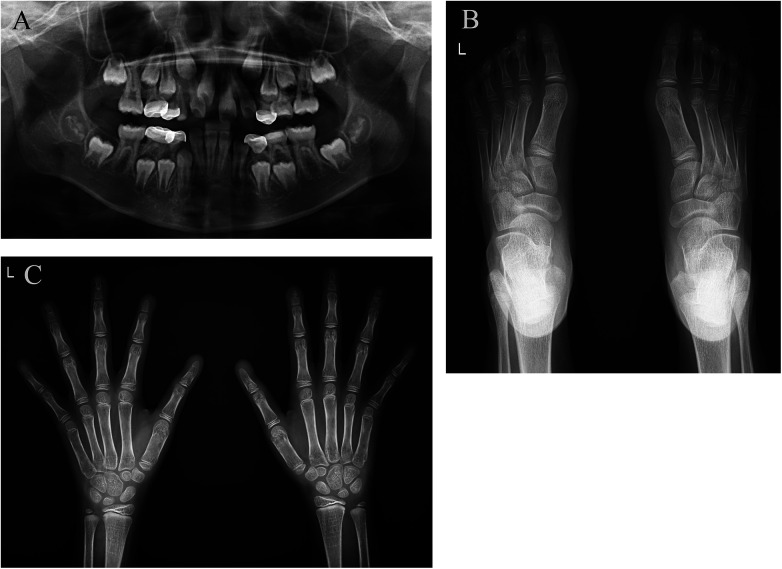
x-ray examination of the patient's teeth, hands, and feet. (A) Shows dental hypoplasia. (B and C) Show that the distal phalanx of both hands and the distal phalanx of both feet become sharp and develop deformities.
